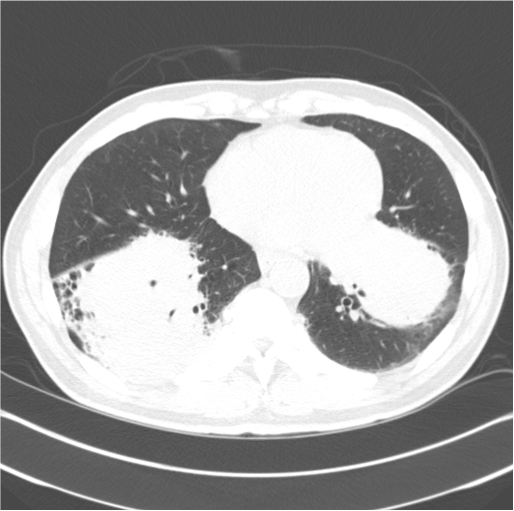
Initial CT scan performed in 2008. CT of thorax without contrast, showing bilateral mass-like opacities.
